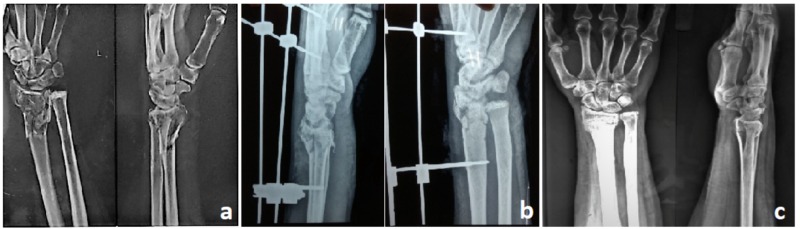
A: Preoperative radiograph showing intraarticular distal radius fracture (AO type C3); B: Postoperative radiograph with external fixator in situ; C: Six-month follow-up radiograph showing radiological union
